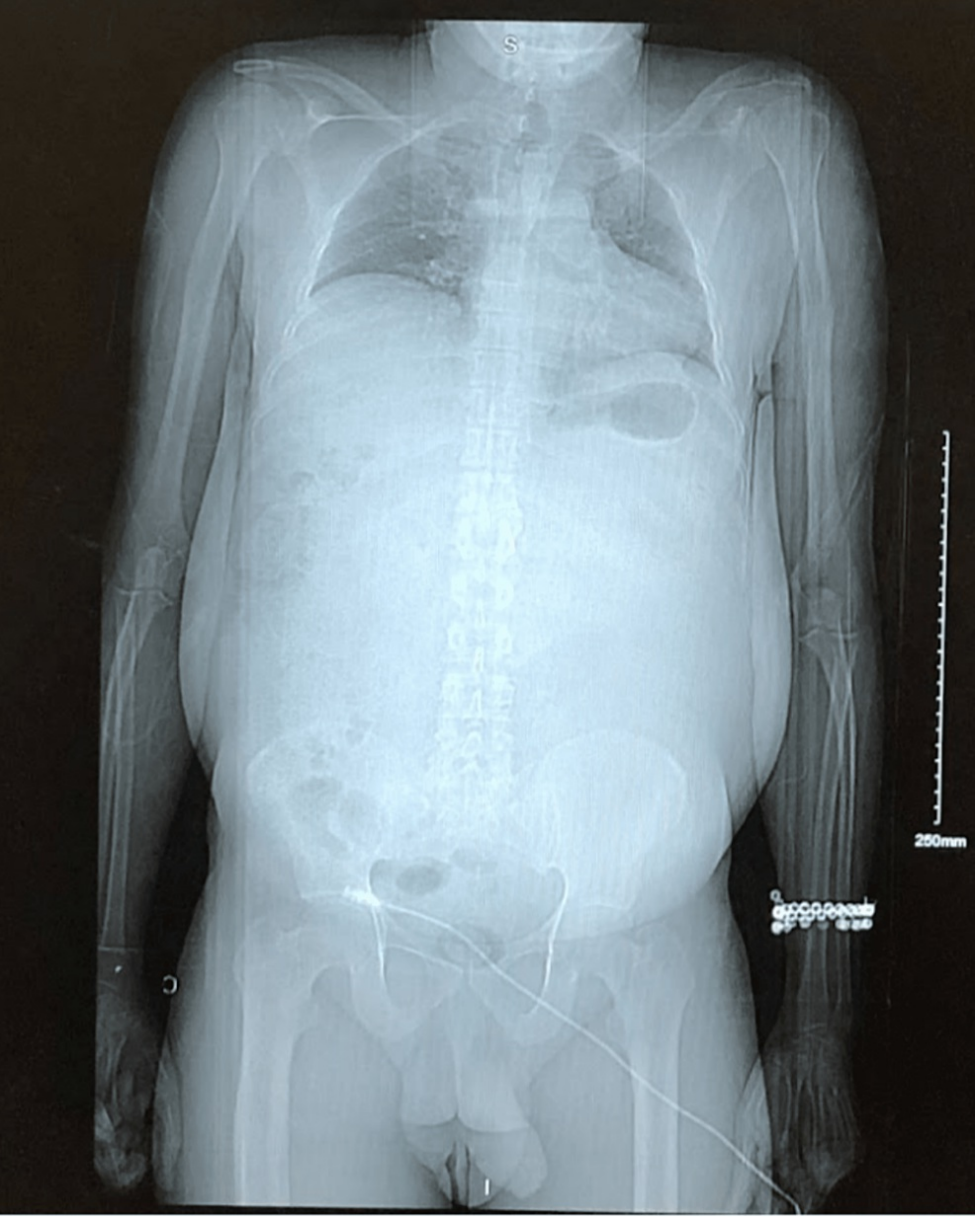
Scout computed tomography of the abdomen without contrast. The patient had a large abdomen relative to the body habitus.
